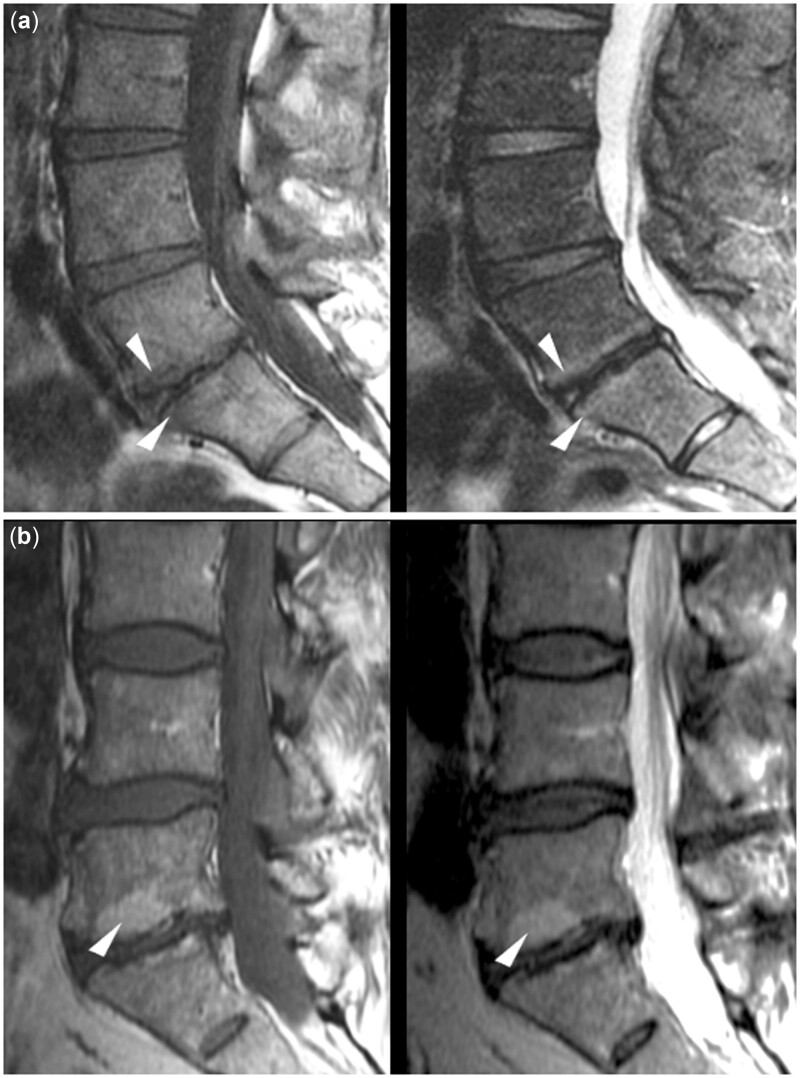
Modic-type endplate changes. Examples of Modic-type endplate changes, which can be identified according to characteristic signal alterations in T1- and T2-weighted images. Modic changes type 1 are typically hypointense on T1- and hyperintense on T2-weighted images (a; arrowheads), and Modic changes type 2 are hyperintense on T1- and hyper- or iso-intense on T2-weighted images without fat suppression (b; arrowheads). Modic type 3 changes are primarily characterized by sclerotic changes (not shown).
